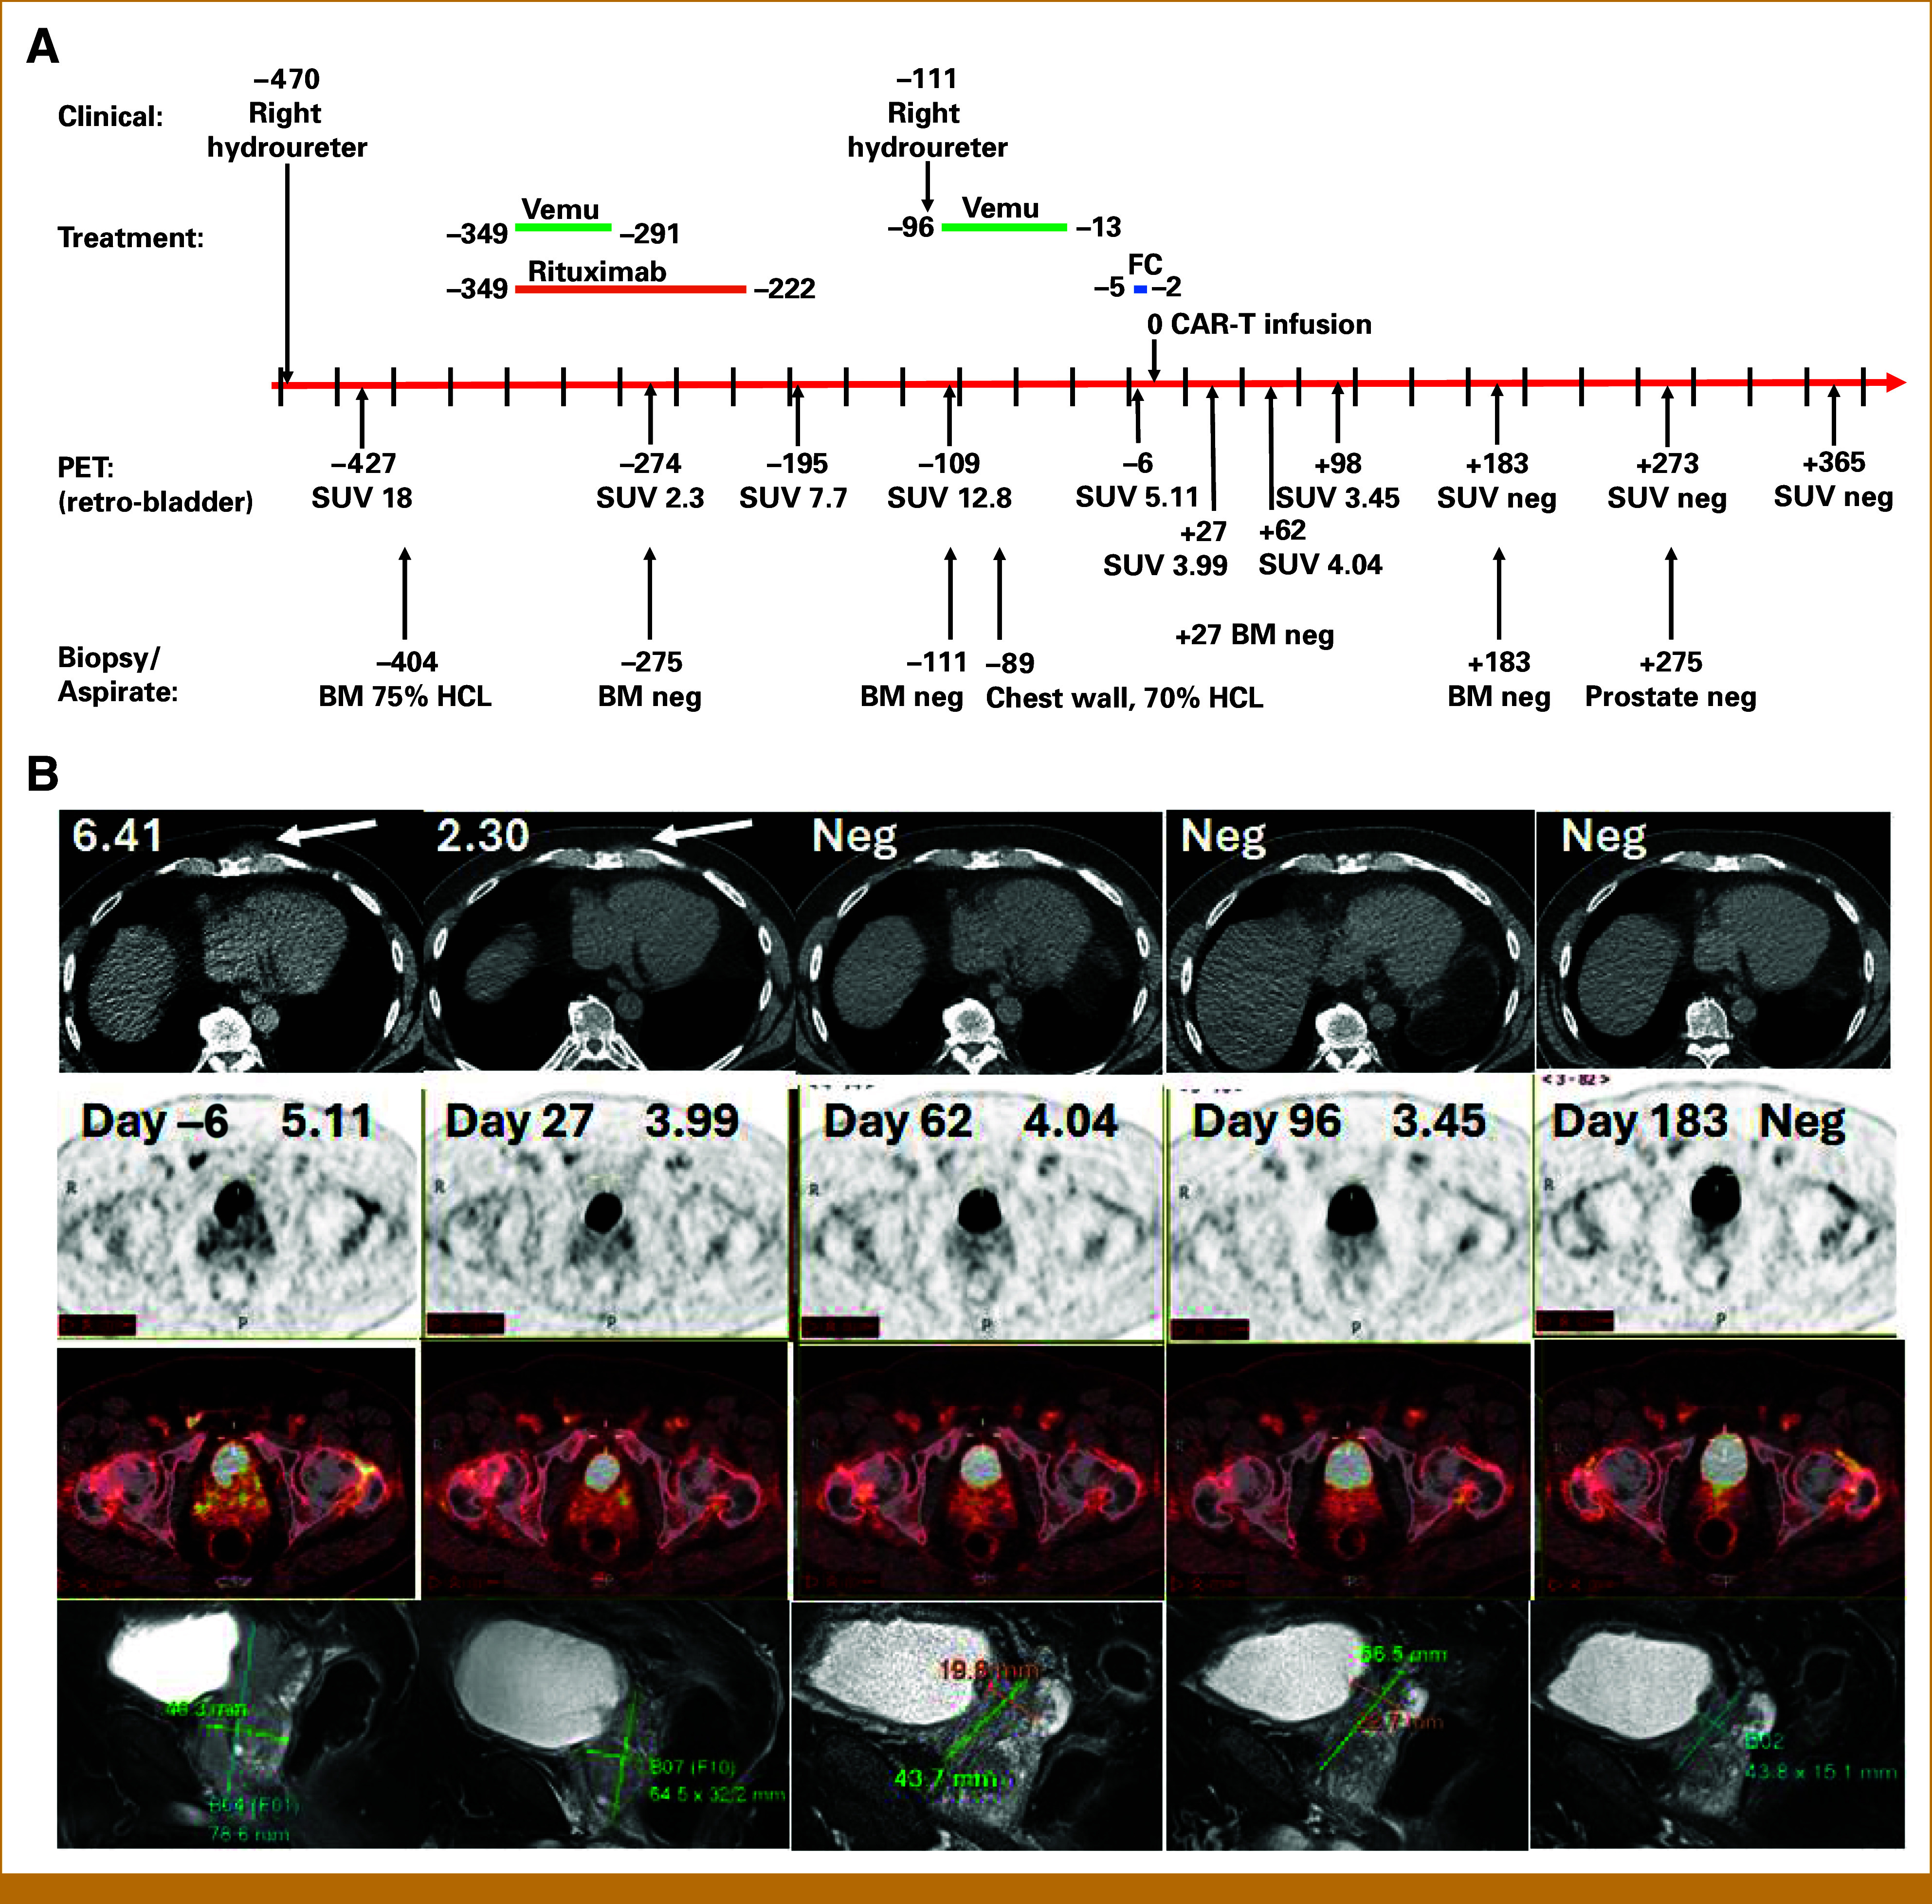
Timeline of treatments and response. (A) Timeline from top to bottom shows clinical presentation of right hydroureter due to posterior bladder (prostate) mass, treatments, PET SUV of posterior (retro-) bladder mass, and biopsy/aspirate of BM, chest wall mass, and posterior bladder (prostate) area. All events include day numbers before (negative) and after (positive) CAR-T infusion on day 0. Start and stop days of Vemu, rituximab, and FC lymphodepleting chemotherapy are shown. Not shown are results of blood flow cytometry to quantify HCL cells, sensitive to 0.002%, which was positive on day +7 but negative on days –160 (when first evaluated at NIH), –111, –69, –34, –6, +14, +20, +27, +62, +97, +183, +273, and +365. (B) PET scan images are shown in the top three rows with day numbers and SUVs. The bottom row shows pelvic MRIs at the same time points as the PET images. Images on days +273 and +365 are not shown but unchanged from +183. BM, bone marrow; CAR-T, chimeric antigen receptor T cell; FC, fludarabine-cyclophosphamide; HCL, hairy cell leukemia; MRI, magnetic resonance imaging; NIH, National Institutes of Health; PET, positron emission tomography; SUV, standardized uptake value; Vemu, vemurafenib.
